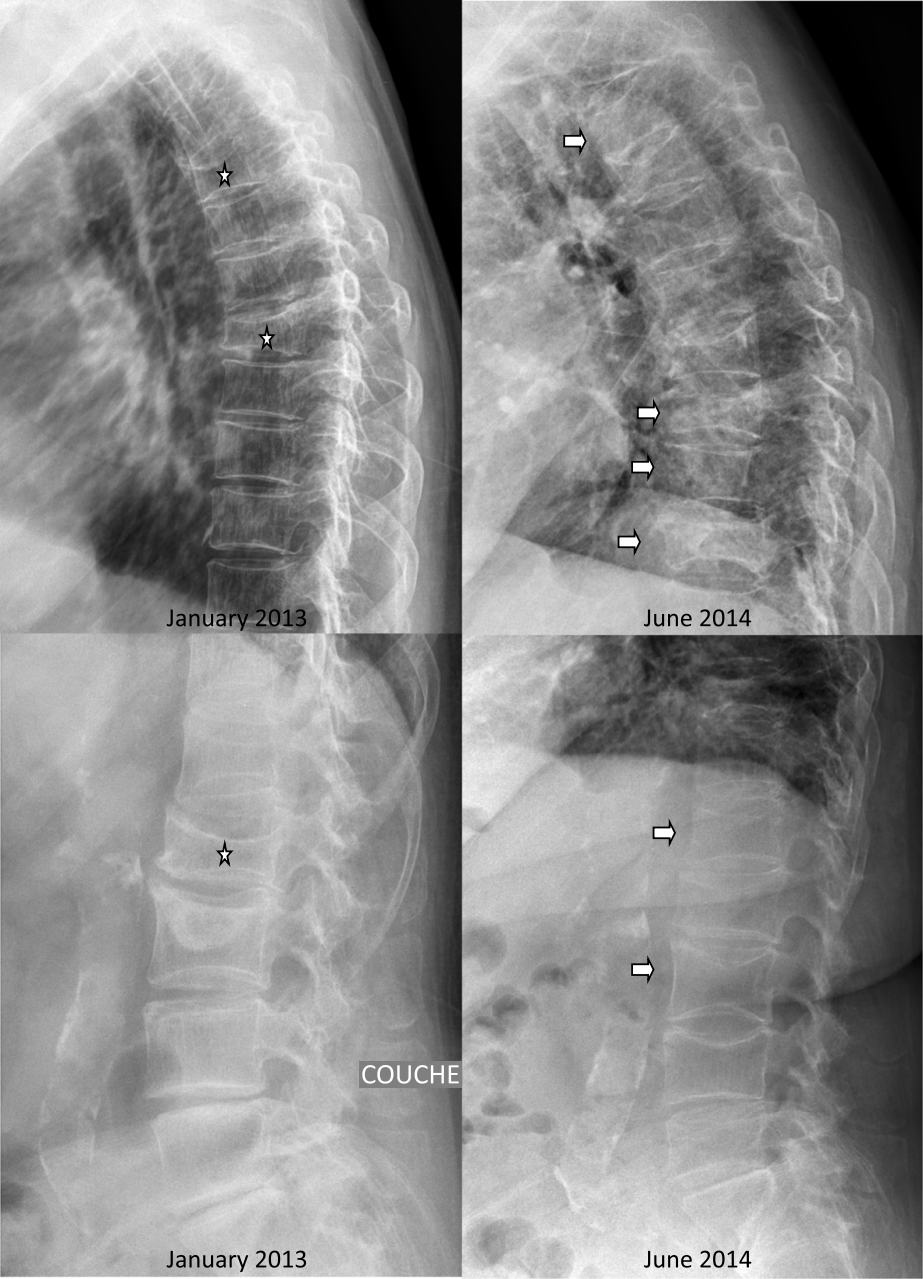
Lumbar and thoracic X-ray in January 2013, 3 months before denosumab was stopped, and in June 2014, 14 months after denosumab was stopped: three old vertebral fractures (L2 in 2004 and T5 T8 L2 (aggravation) during the period 2005-2008)(stars) and 6 new fractures (T4 T9 T10 T11 L1 L3)(arrows) with denosumab rebound. Stars are the old fractures and arrows the new one
